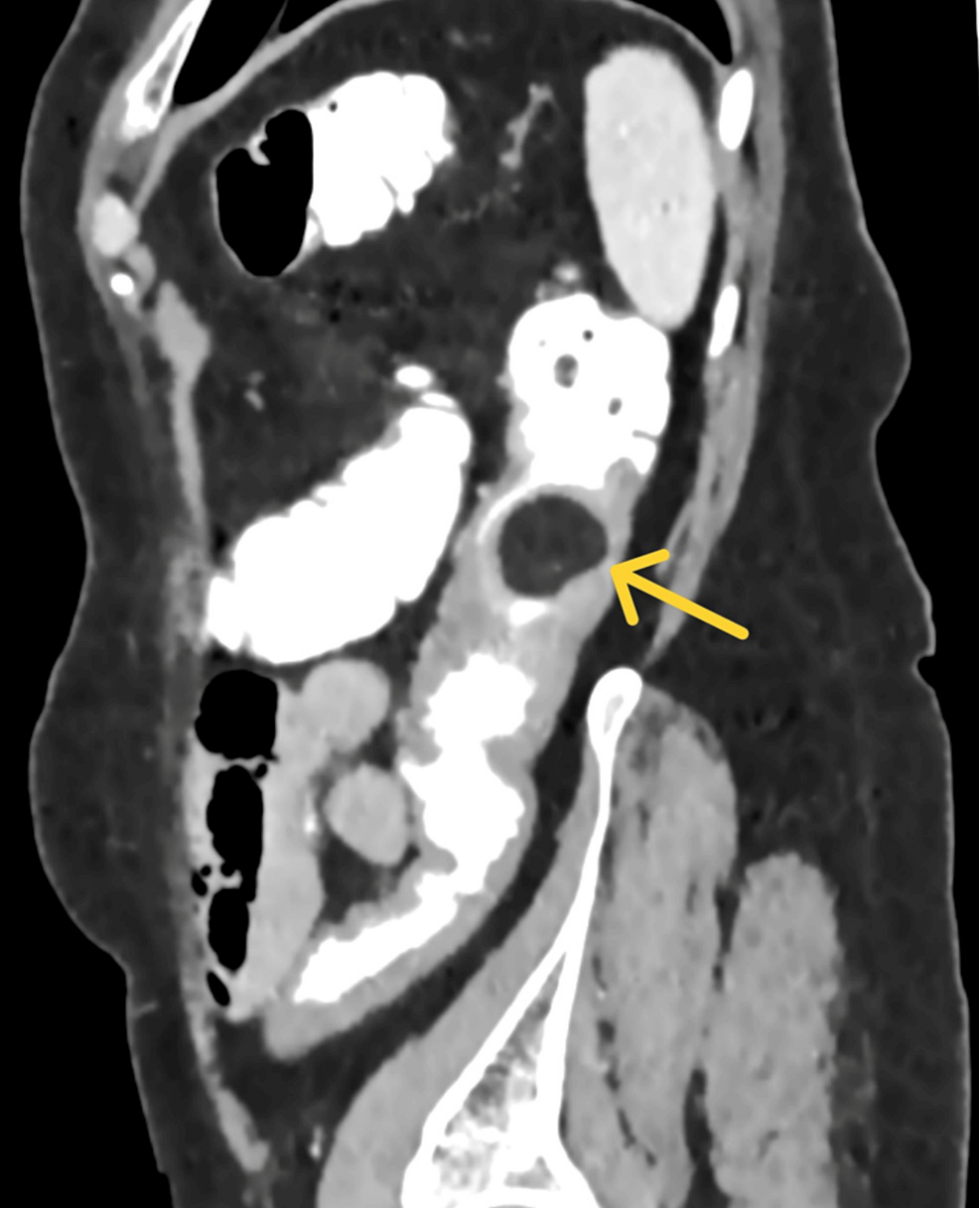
Contrast-enhanced abdominal computed tomography. Sagittal plane. A colonic lipoma (yellow arrow) is causing significant luminal obstruction. Diffuse thickening of the descending colon over a 6-centimeter length.
